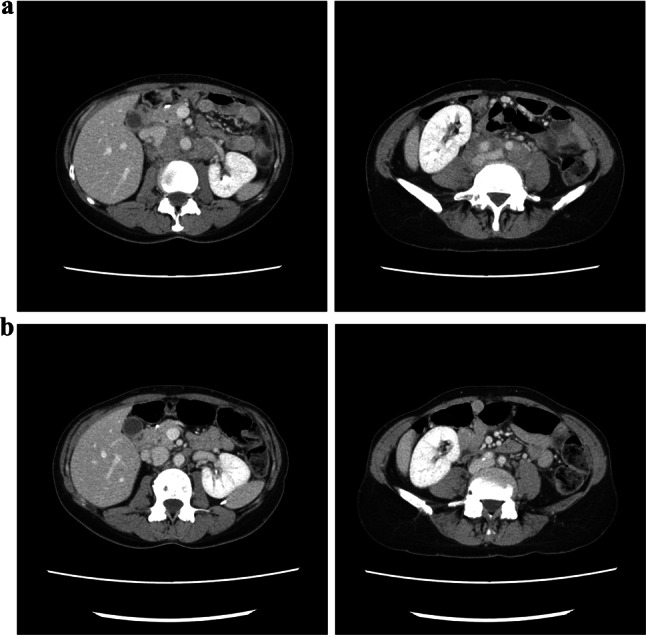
Abdominal CT image prior to treatment (a) and after the second infusion of trastuzumab (b). The para-aortic lymphadenopathy shrunk remarkably after treatment
